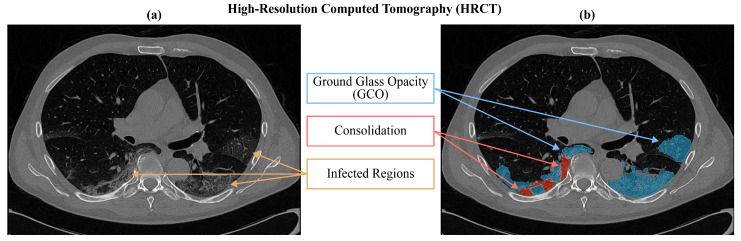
Manifestations of COVID-19-infected regions on an HRCT of a confirmed patient. Panel (a) shows the original grey-level intensities, while in Panel (b) the infected regions are manually enhanced by radiologists (GGO in blue and Consolidation in red).
